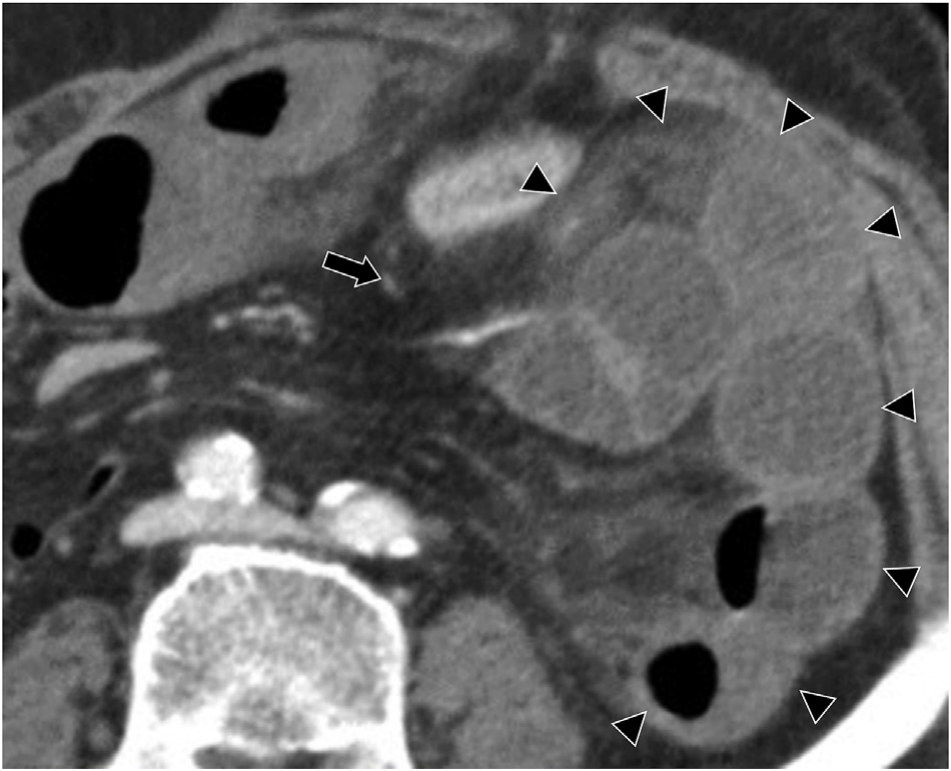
Axial computed tomography (CT) of Case 3 showing dilated and edematous small bowel (arrowheads). It also shows passage of the small bowel through the defect behind the superior rectal artery (SRA; arrow).
